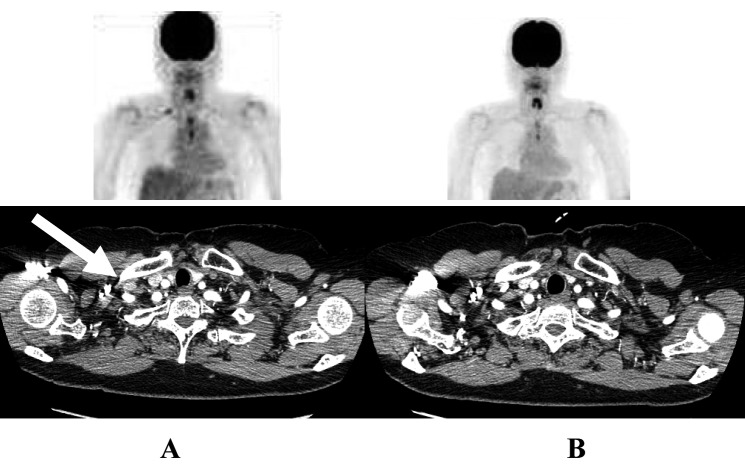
The response to second-line ADOC chemotherapy in case 1. Chest computed tomography and positron emission tomography before initial ADOC chemotherapy (A) and after four cycles of second line ADOC chemotherapy (B).
